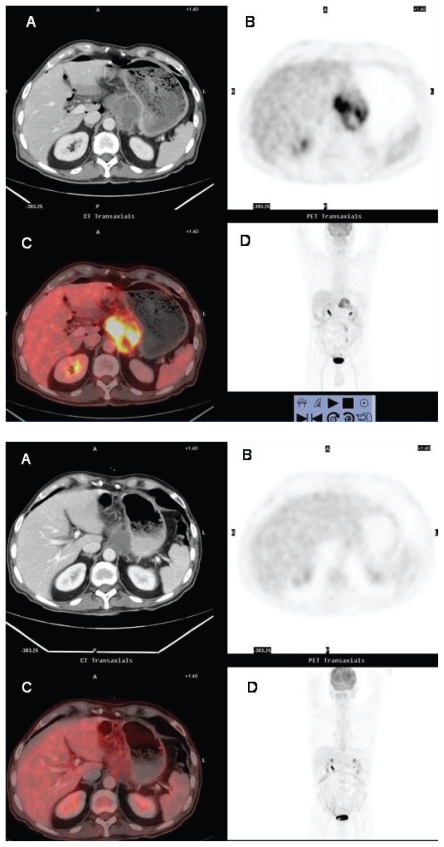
Assessment fo residual mass at completion of initial treatment in lymphoma. 9A: Pretreatment ^18^FDG PET/CT study of a 27 year-old man with DLCB NHL. There is an abdominal mass that exhibits intense ^18^FDG uptake, consitent with lymphoma 9B: Postreatment ^18^FDG PET/CT study shows persistent, though decresed in size abdominal soft-tissue mass, and complete resolution of the abnormal metabolic activity. The patient has remained free of relapse.
